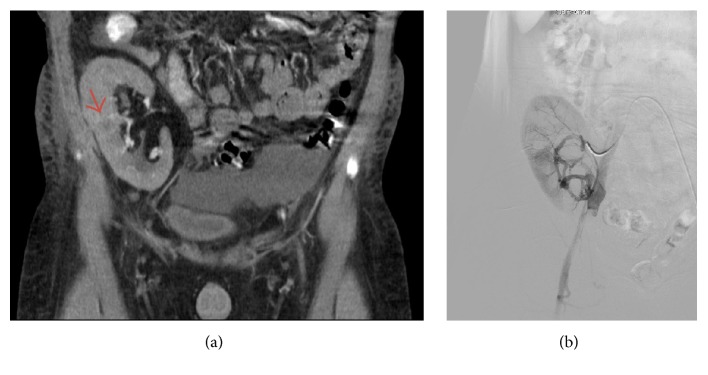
(a) Computed tomography scan coronal section demonstrates an enhancing, round lesion, suspicious for malignancy in the mid renal zone (arrow). (b) Embolization of the renal artery of the renal allograft was performed before graft nephrectomy.
